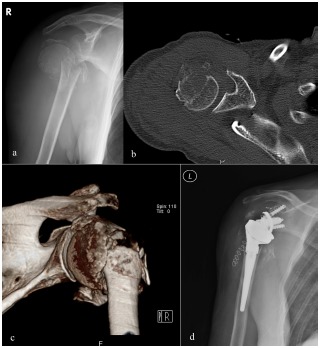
X-Ray (a), CT (b) and 3D reconstruction CT (c) of a 4-part humeral head fracture treated with reverse shoulder arthroplasty.
